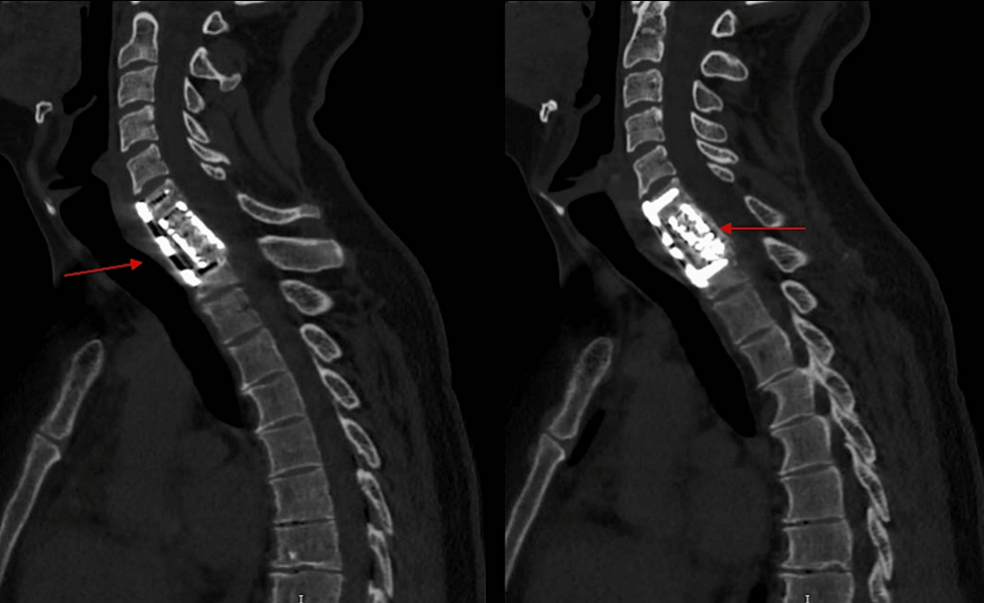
CT Cervical sagittal views at two years follow up
